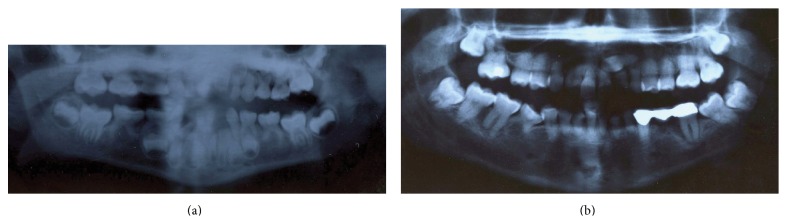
Dental X-rays performed at the age of 6 (a) and the age of 19 (b). The lower second bicuspids are absent. There are two supernumerary teeth underneath her secondary lower incisors, which may represent ectopic, malformed bicuspids.
